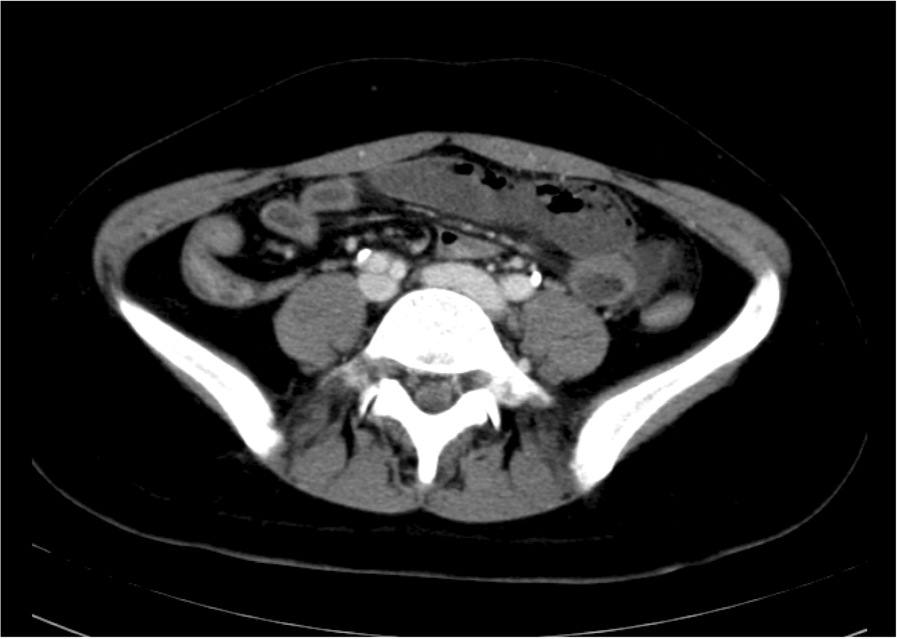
Findings on abdominal contrast-enhanced computed tomography. Poor contrast enhancement of the small intestine is noted
